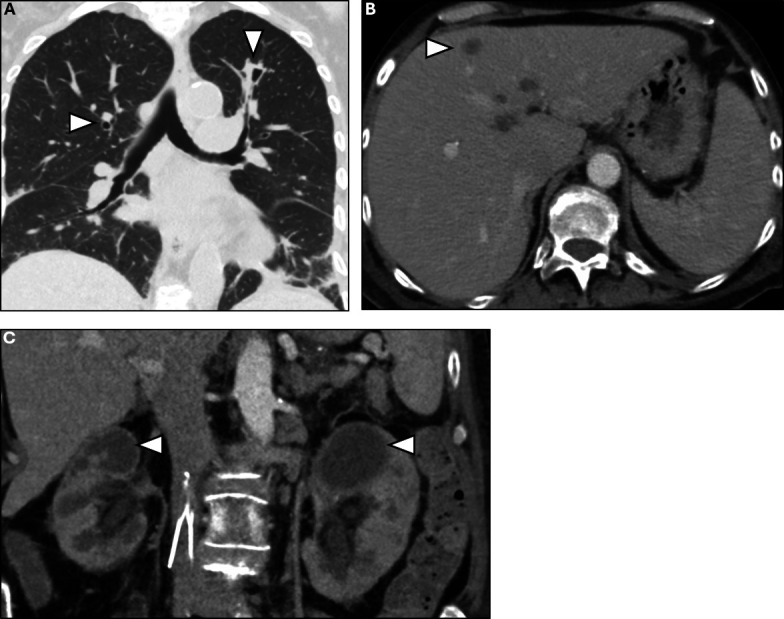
Non-contrast CT of the chest demonstrating reduction in bilateral cavitary infiltrates (A). Contrast CT of the abdomen and pelvis demonstrating stable hypodensities in the liver and kidneys (B and C).
